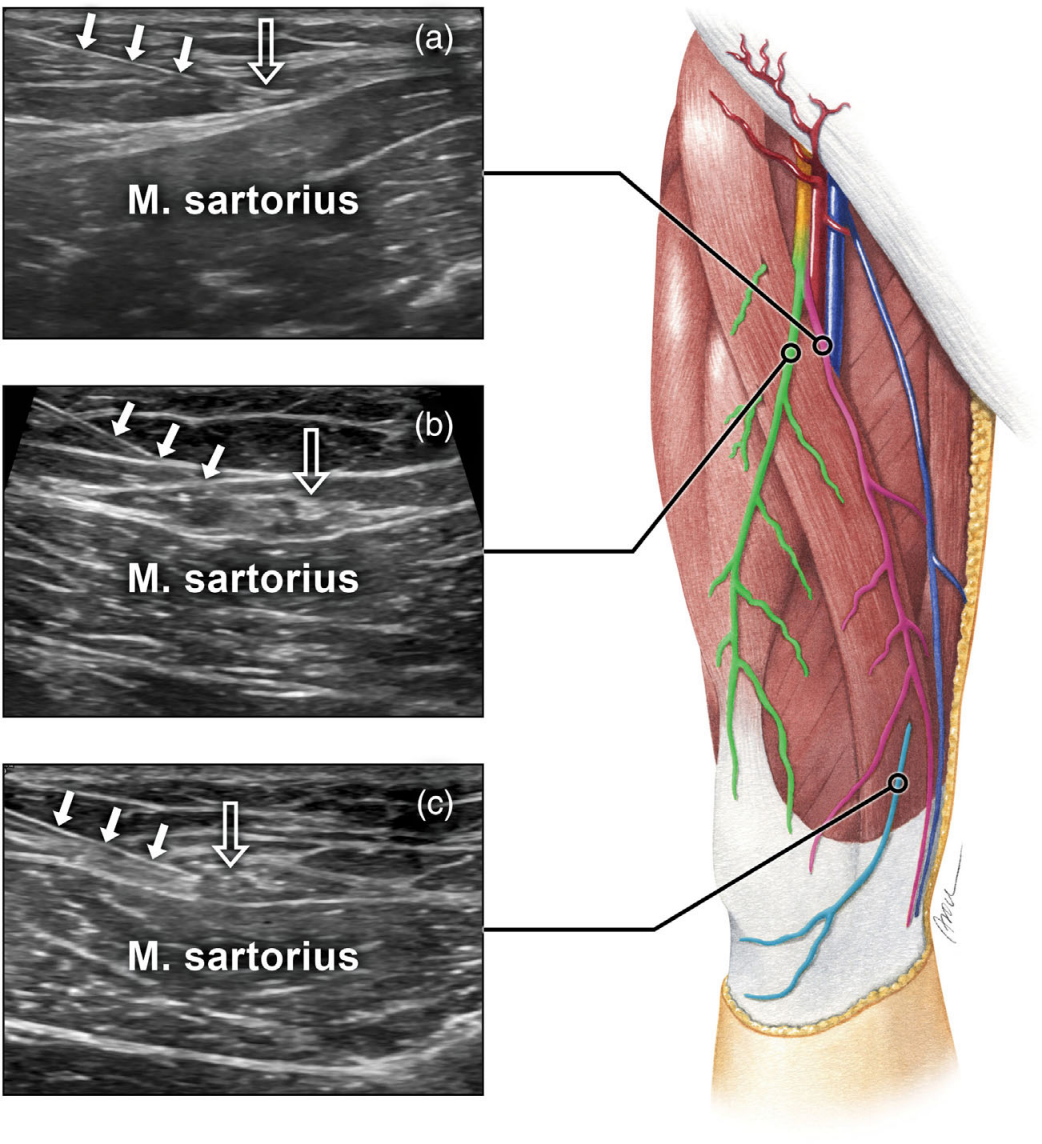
Examples of ultrasound‐guided nerve blockades of the intermediate femoral cutaneous nerve (a), the medial femoral cutaneous nerve (b), and the infrapatellar branch of the saphenous nerve (c) [Color figure can be viewed at wileyonlinelibrary.com]
